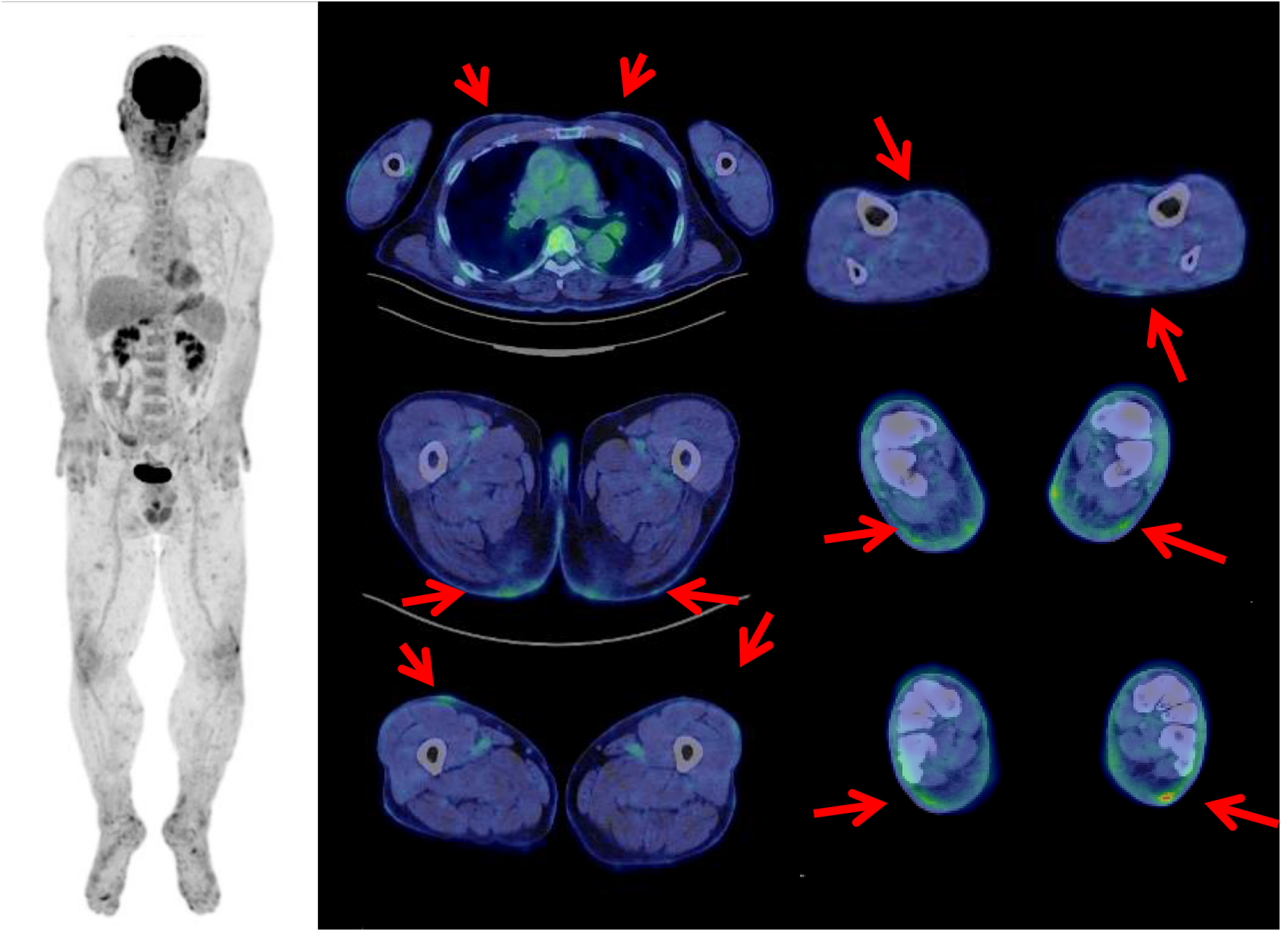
A 64-year-old male suffered rash-like skin redness, slightly raised or scaly round patches on the skin of the face, trunk and extremities. The biopsy of the skin lesion revealed the diagnosis of cutaneous peripheral T cell lymphoma. Whole-body FDG PET maximum projection and cross-sectional images showed FDG uptake (SUVmax 4.5) in the systemic skin lesions (arrows)
